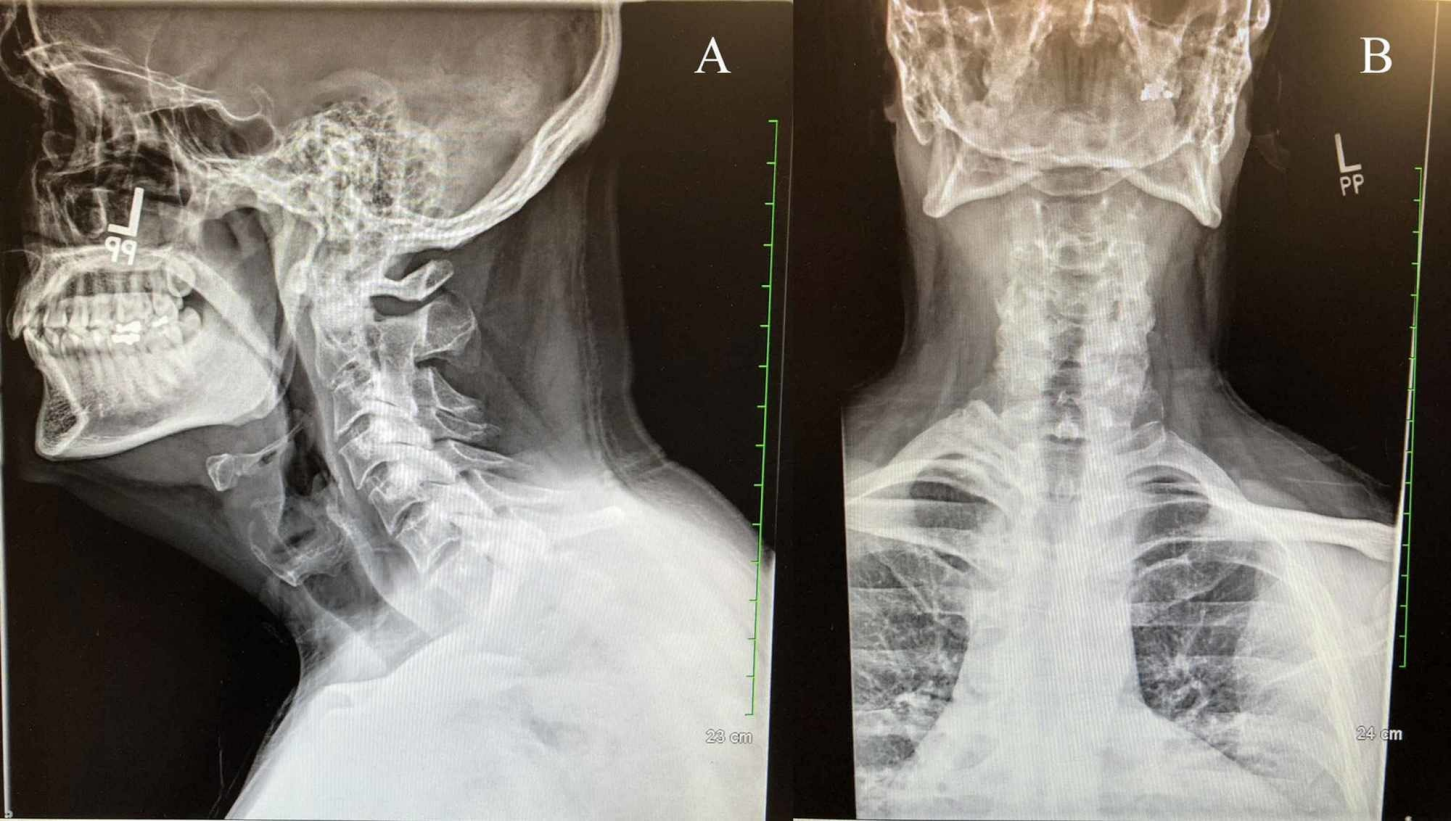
X-ray of the cervical spine Findings: The heights of the cervical vertebral bodies are normal. There is the mild narrowing of the cervical C2-C3 disc space. There is a mild anterior spurring at the cervical C3-C4 disc levels. There does not appear to be significant narrowing. Remaining cervical disc spaces are fairly well-preserved. There are mild degenerative facet changes in the mid and upper cervical spine. The odontoid appears intact. Impression: Mild degenerative disc changes. Degenerative facet changes. There is no evidence of cervical spine fracture.
